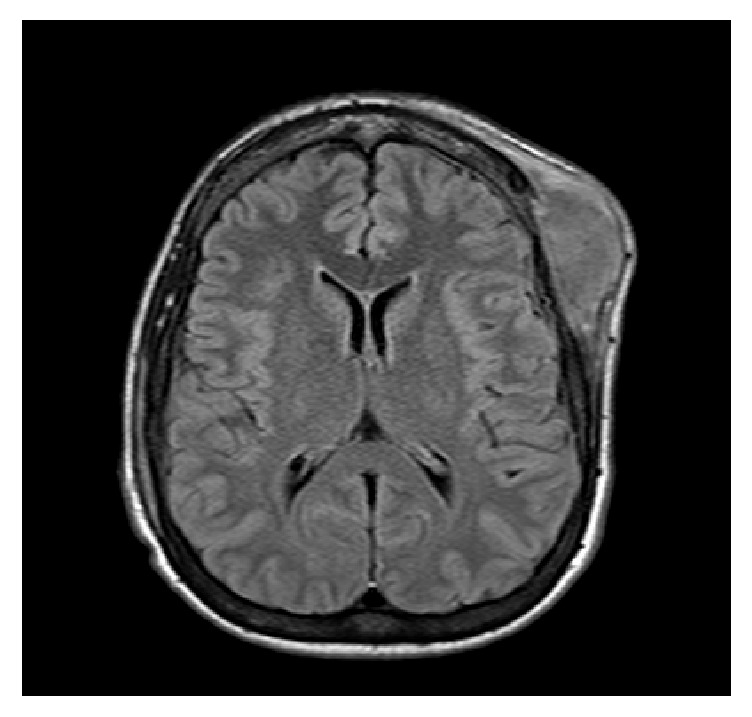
MRI of the brain displaying left frontoparietal dominant extracranial soft tissue lesion.
